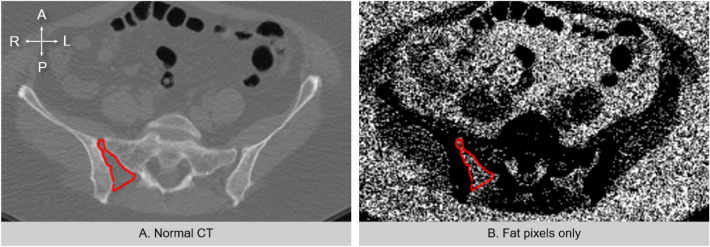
Computed tomography (CT) results when applying the Hounsfield unit range in VolSEQ (− 150 to − 50 HU), axial view. (A) Normal CT with the posterior sacroiliac region (PSIJ) highlighted in red. The posterior boundary is an arbitrary line running between ilium and sacrum (B) The same slice with only pixels of fat (in white). A: anterior, P: posterior, L: left, R: right.
